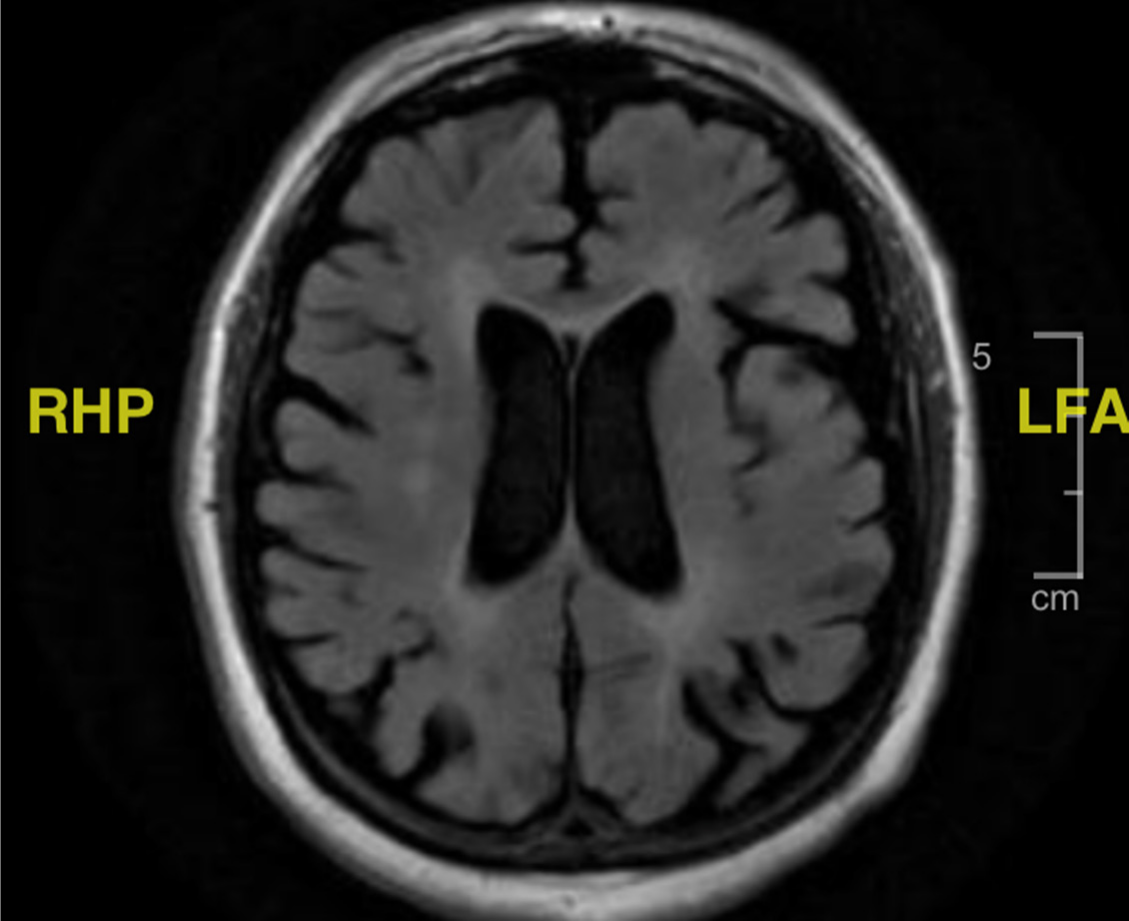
Brain MRI showing chronic periventricular demyelination and no acute intracranial pathology.
